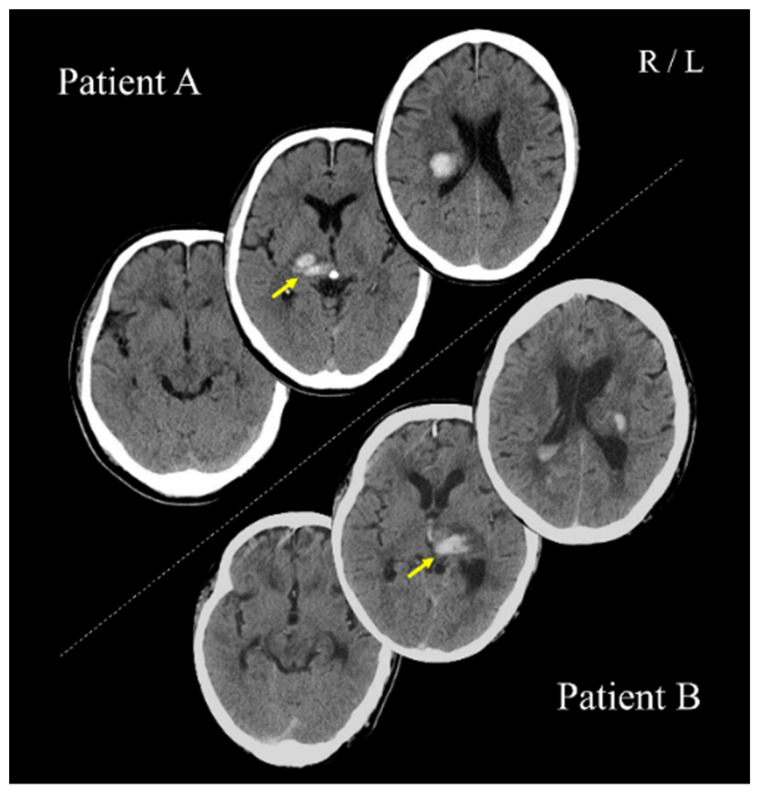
Computed tomography scans show the lesion sites of patients A and B. These images were acquired within a day after stroke onset. Yellow arrows indicate the lesion sites. R: right hemisphere; L: left hemisphere.
